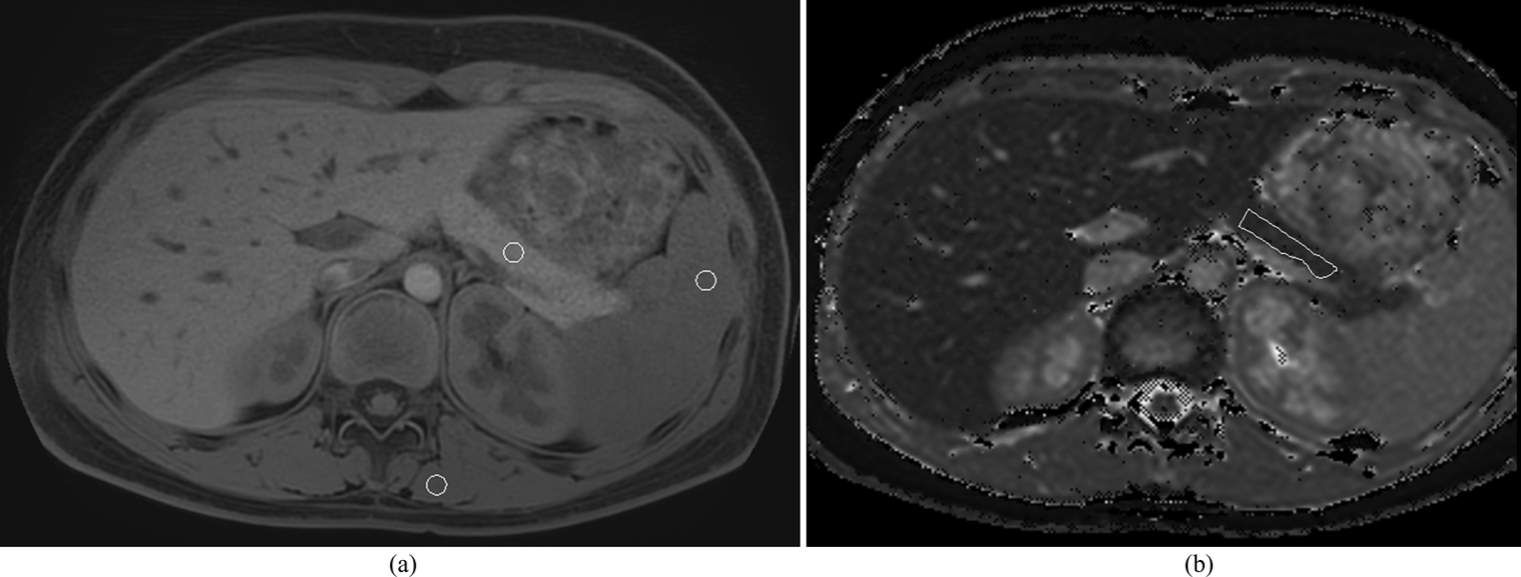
Axial 1.5T (A) T1-weighted 3D VANE and (B) T1 parametric map images in a 14-year-old boy with no pancreatic disease. Circular regions of interest (ROIs) in the pancreas, spleen, and paraspinal muscle on T1-weighted 3D VANE images were used to calculate T1-weighted signal intensity ratios (T1 SIR). An irregular ROI drawn on the pancreas on the corresponding slice on the T1 parametric map was used to measure estimated T1 relaxation time. ROIs are indicated using a white outline. Pancreas:spleen T1 SIR was 1.48 and Pancreas:paraspinal muscle T1 SIR was 1.70 with corresponding T1 relaxation time of 658 msec
